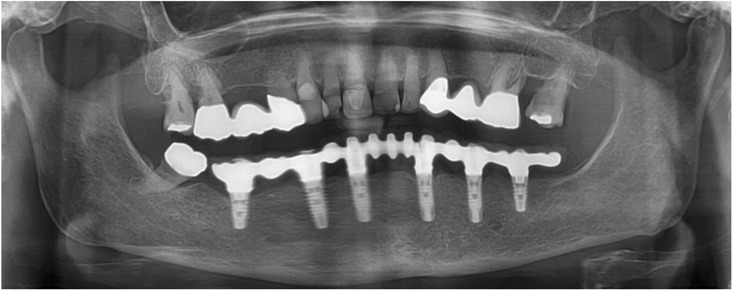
Panoramic radiograph at the 12-months recall appointment
